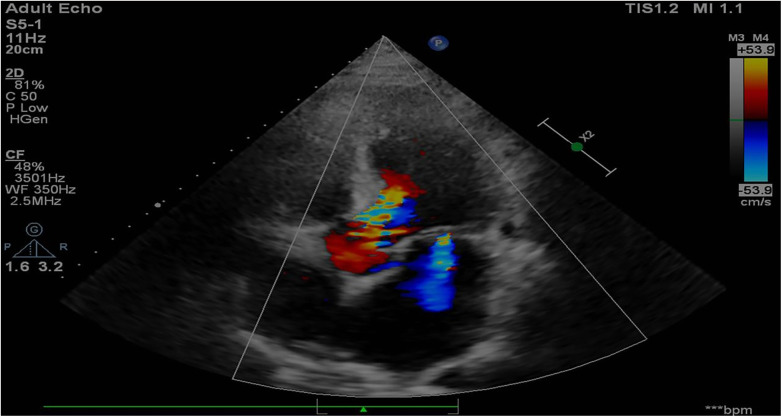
Echocardiogram conducted at the time of admission showing global cardiac dilation and moderate aortic regurgitation.
